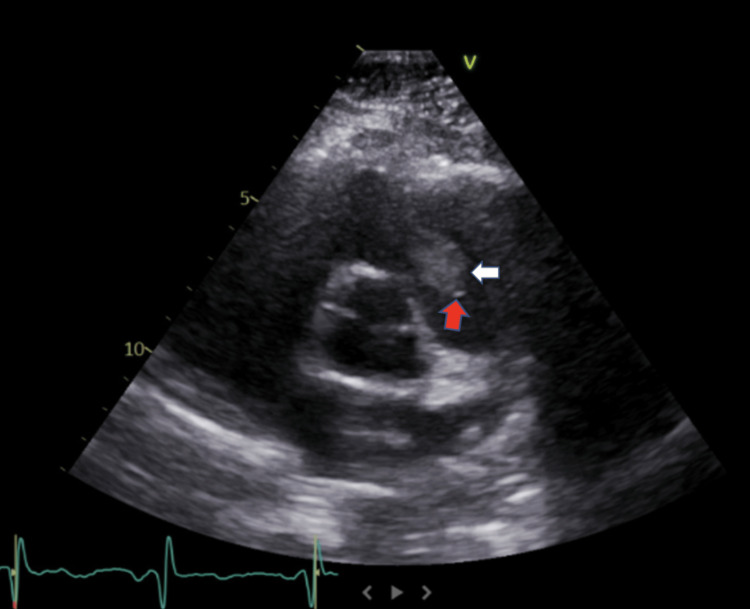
Parasternal long-axis view echocardiography of the right ventricle The white arrow shows the thrombus found in the right ventricle attached to the pulmonic valve (red arrow).
